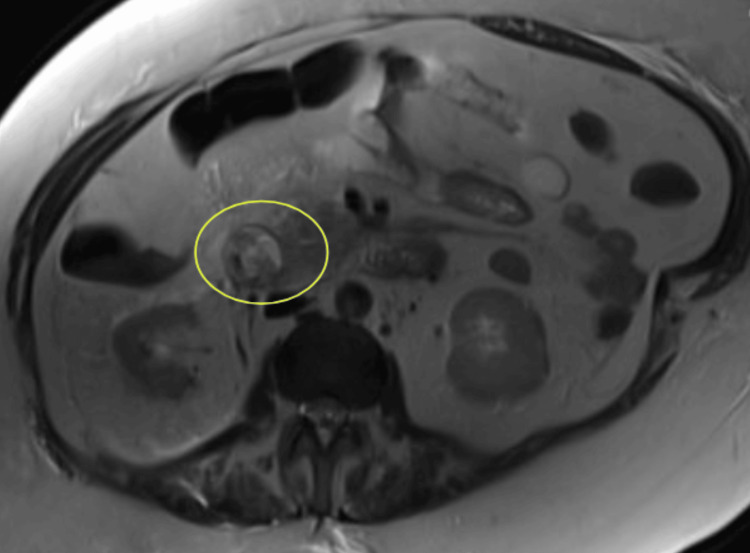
MRI of the abdomen revealing a small amount of fluid (circled in yellow) in the right anterior pararenal space in the center of the pancreatic head.
